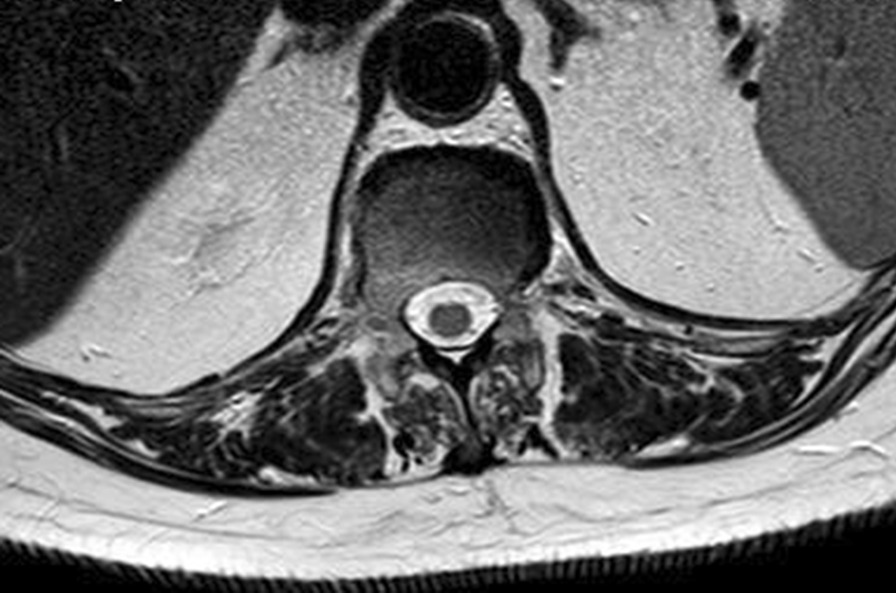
Axial T2 magnetic resonance imaging showing a complete resolution of the paravertebral abscess that was originally adjacent to T12
